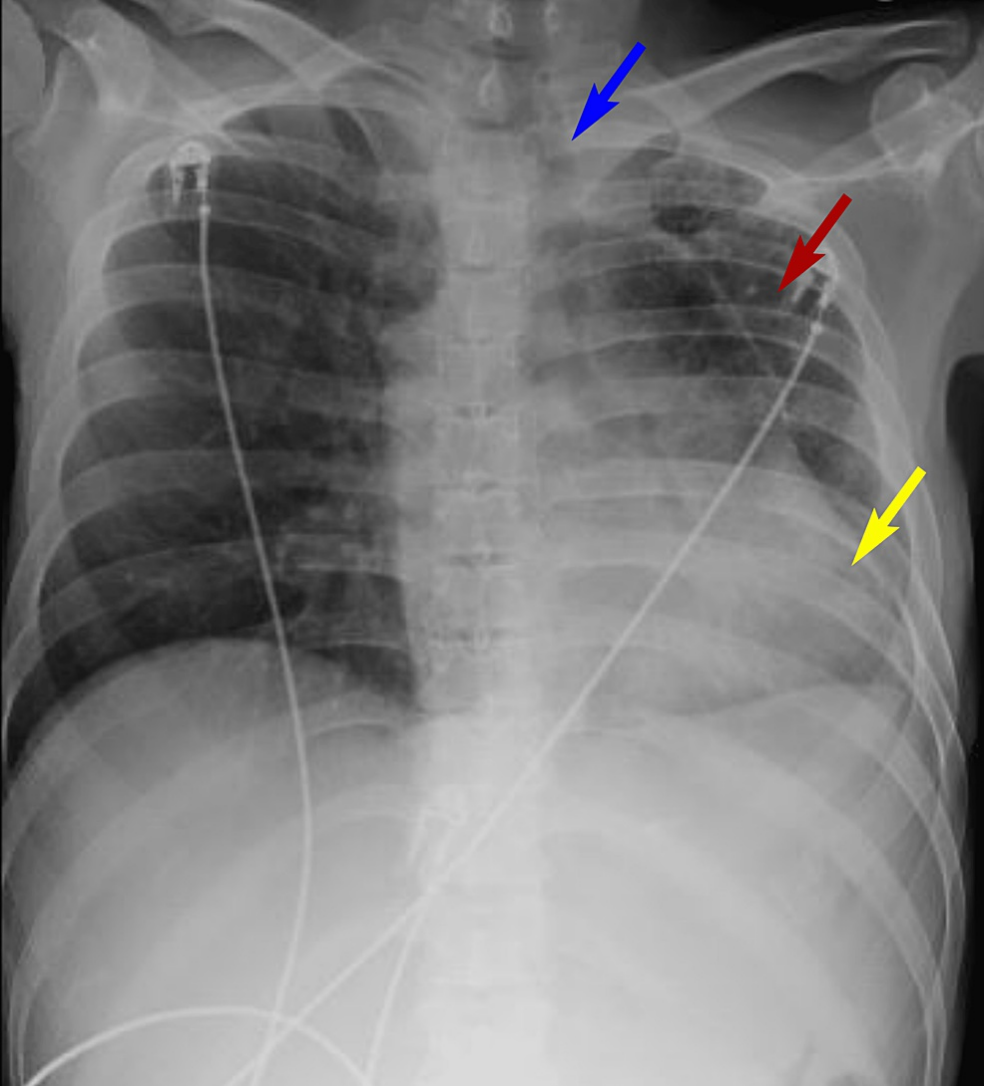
Chest radiograph, posterior-anterior view Chest radiograph posterior-anterior view shows a hypoplastic left lung (red arrow) with right-to-left tracheal deviation (blue arrow) and a prominent cardiac silhouette (yellow arrow) overlying the left thorax.
